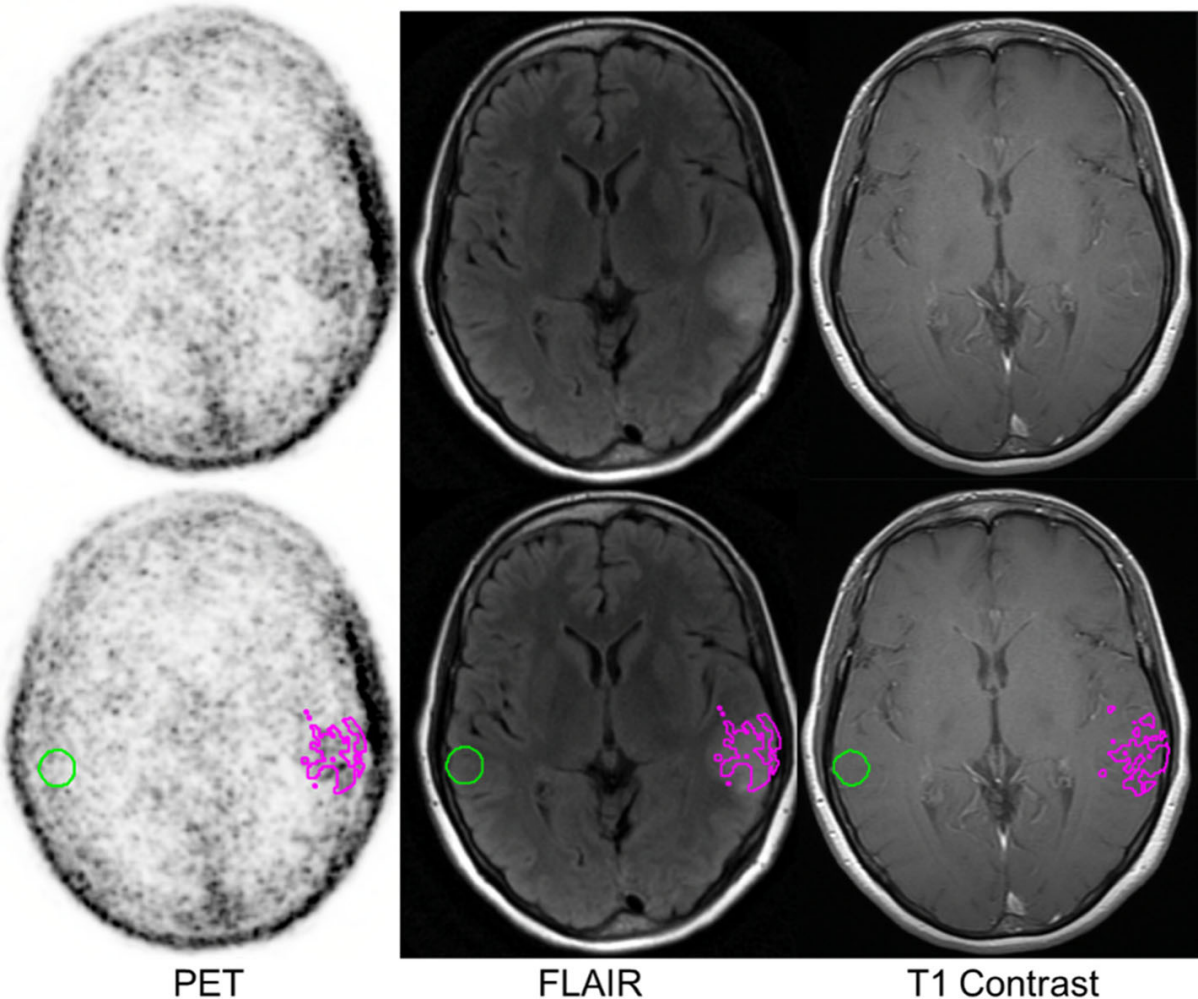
Fluciclovine PET at 30 min post-injection of oligodendroglioma with regions overlaid on MRI. Green sphere is the area of normal brain fluciclovine uptake. Magenta area is the metabolic tumor uptake defined as 1.3* contralateral normal brain uptake (TB_mean1.3_)
